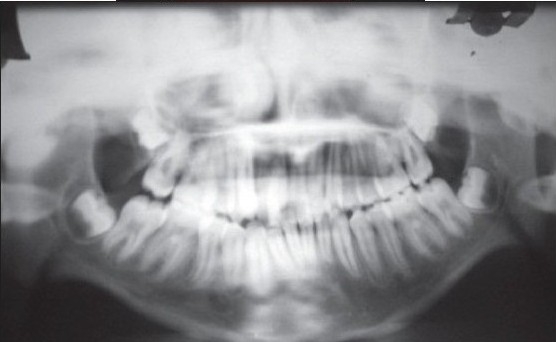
Preoperative orthopantomogram showing bilateral temporomandibular joint ankylosis
